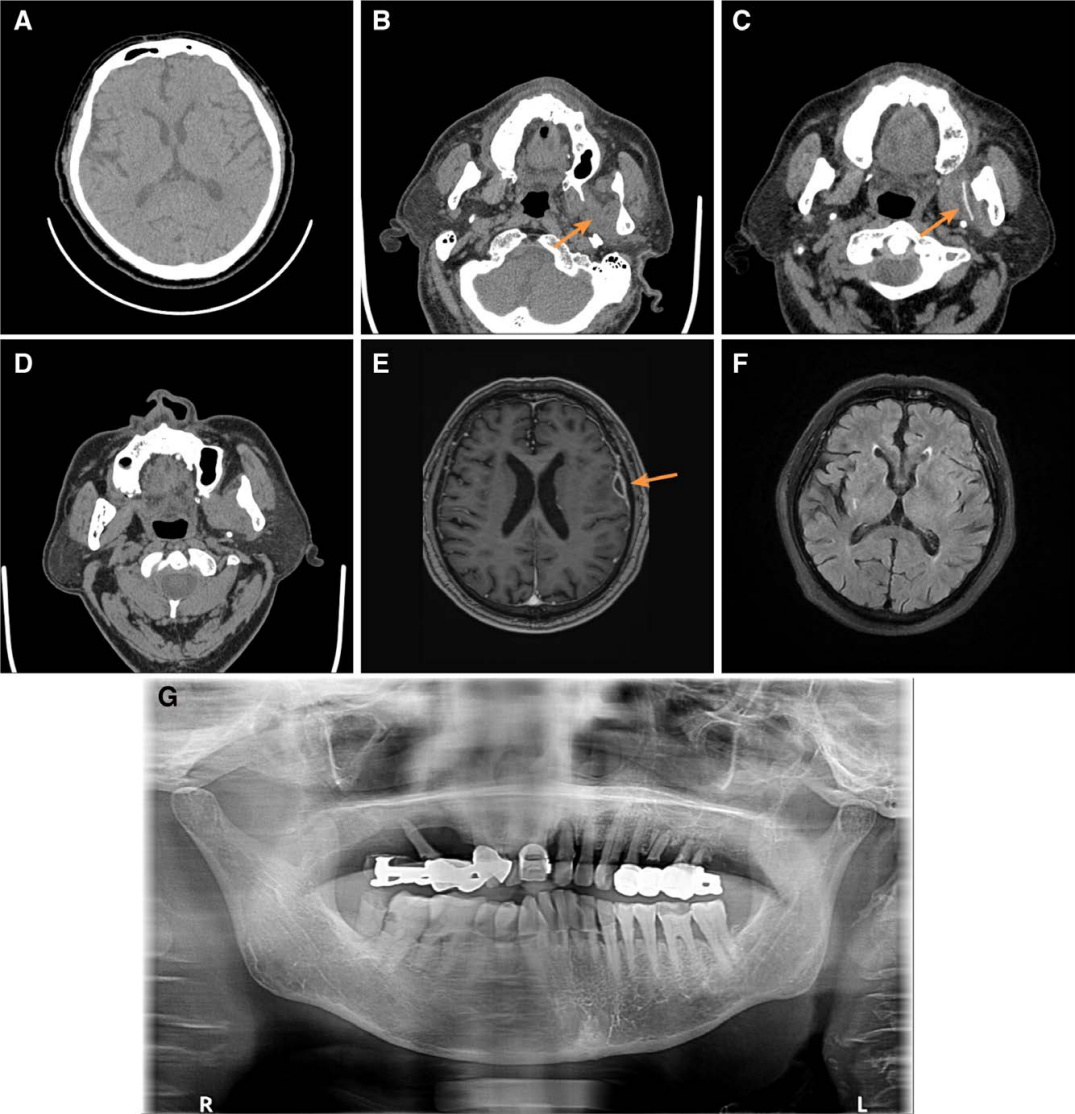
Imaging and clinical course of case 1. (A) Brain CT on admission. (B) Swelling of ectopterygoid muscle (arrow). (C) Incision and drainage with drain placement (arrow indicates drainage tube). (D) Review after drain removal. (E) Brain abscess formation (arrow). (F) Follow-up cranial MRI after 3 months. (G) Intraoral dental condition. CT = computed tomography.
